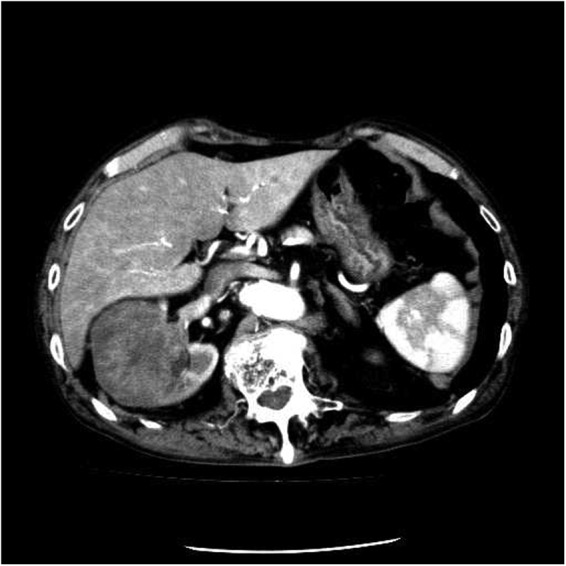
Enhanced CT scan of case 2 demonstrated a right renal tumor. The tumor was diagnosed as RCC by the radiologist who is the attending doctor and belongs to the Japan Radiological Society. RCC, renal cell carcinoma.
